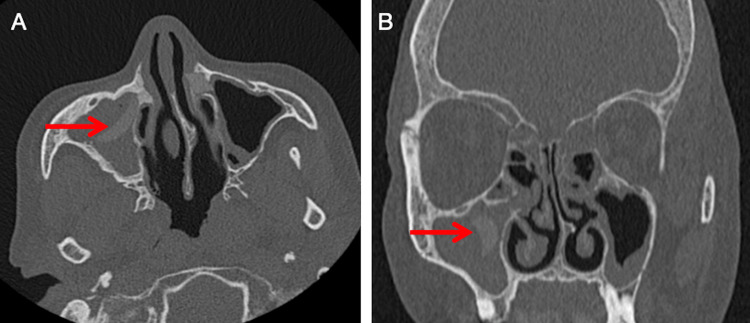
Referenced in Case 1, Diagnostic Assessment section - axial (A) and coronal computed tomography (B) scans showing a hyperdense foreign body (red arrow) in the right maxillary sinus.
